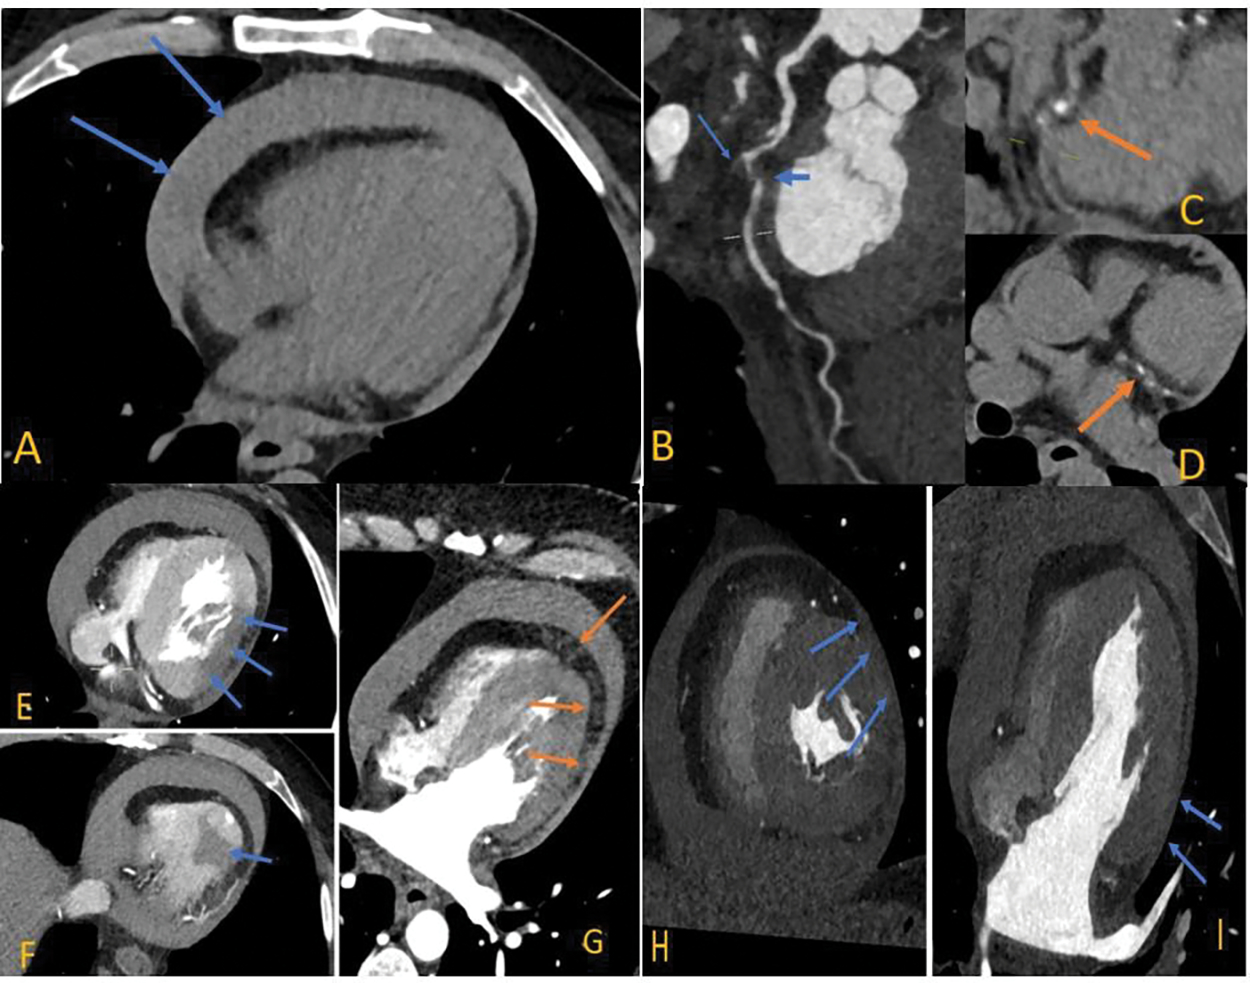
Multi-parametric CT imaging of left ventricular free wall rupture.
